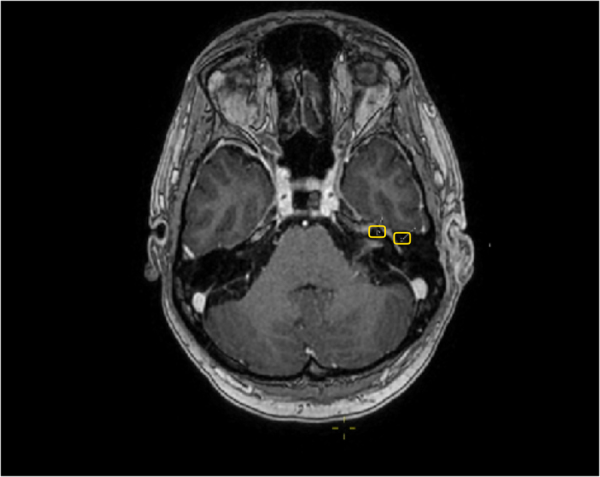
Magnetic resonance image of internal auditory meatus
